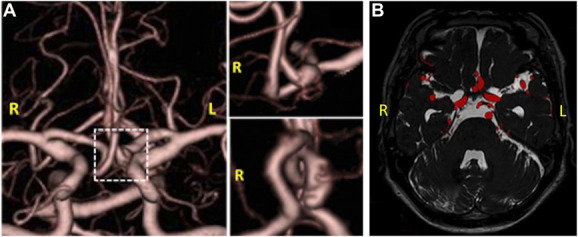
A, Follow-up computed tomography angiography revealed the left A2 aneurysm and presumptive failed A3-A3 bypass. B, Fusion MRI image confirmed the aneurysm and no sign of thrombosis.
